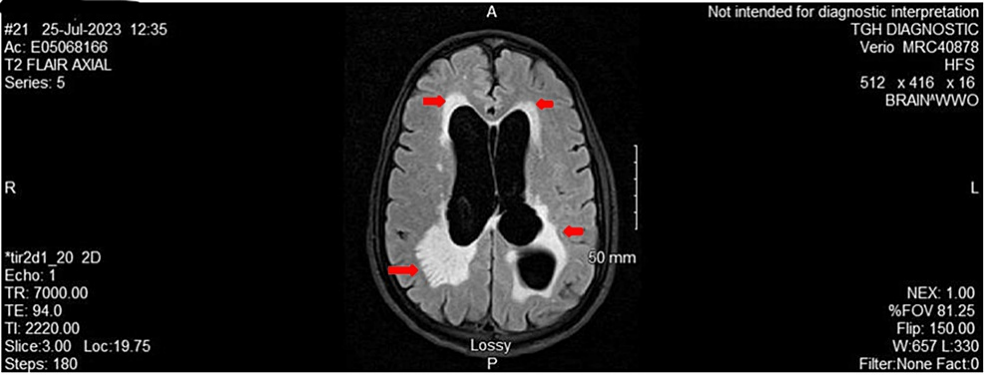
MRI brain: T2 FLAIR axial The image shows bilateral periventricular white matter hyperintensities FLAIR: fluid attenuated inversion recovery; MRI: magnetic resonance imaging
